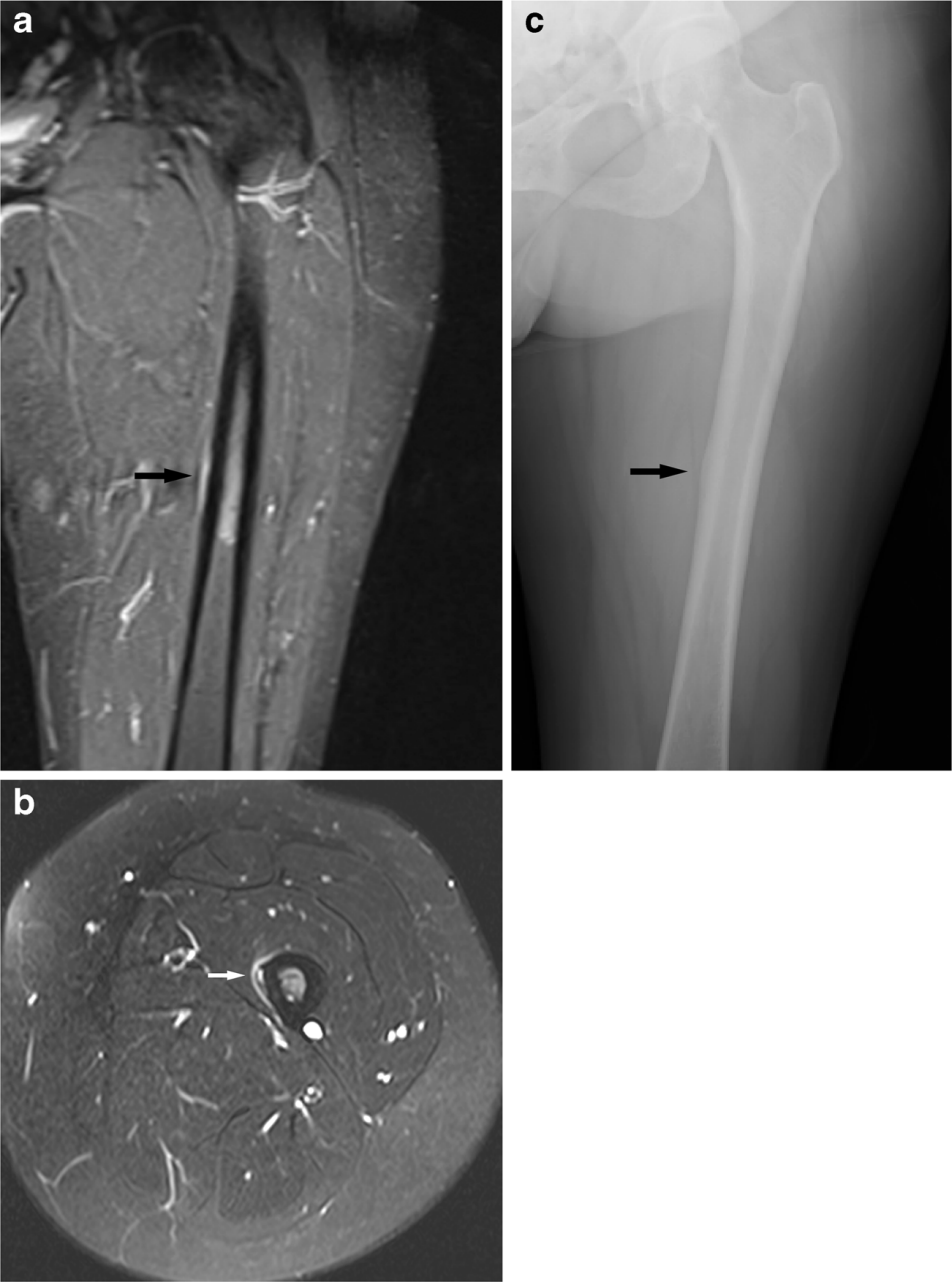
Typical stress lesion of the left midfemoral shaft in a middle-aged female. This is difficult to appreciate on the coronal images (a, short tau inversion recovery, STIR, image) and is just visible as subtle medial high fluid signal at the midshaft (black arrow). It is best seen on an axial proton density fat saturation (PD-FS) sequence (b) as a medial periosteal reaction and intracortical high fluid signal (white arrow). This was not visible on a radiograph taken at the same time but became radiographically visible 2 months later (c) as a subtle periosteal reaction medially (black arrow). Typical femoral stress fractures are located on the medial femoral shaft and have a broad-based bone reaction
